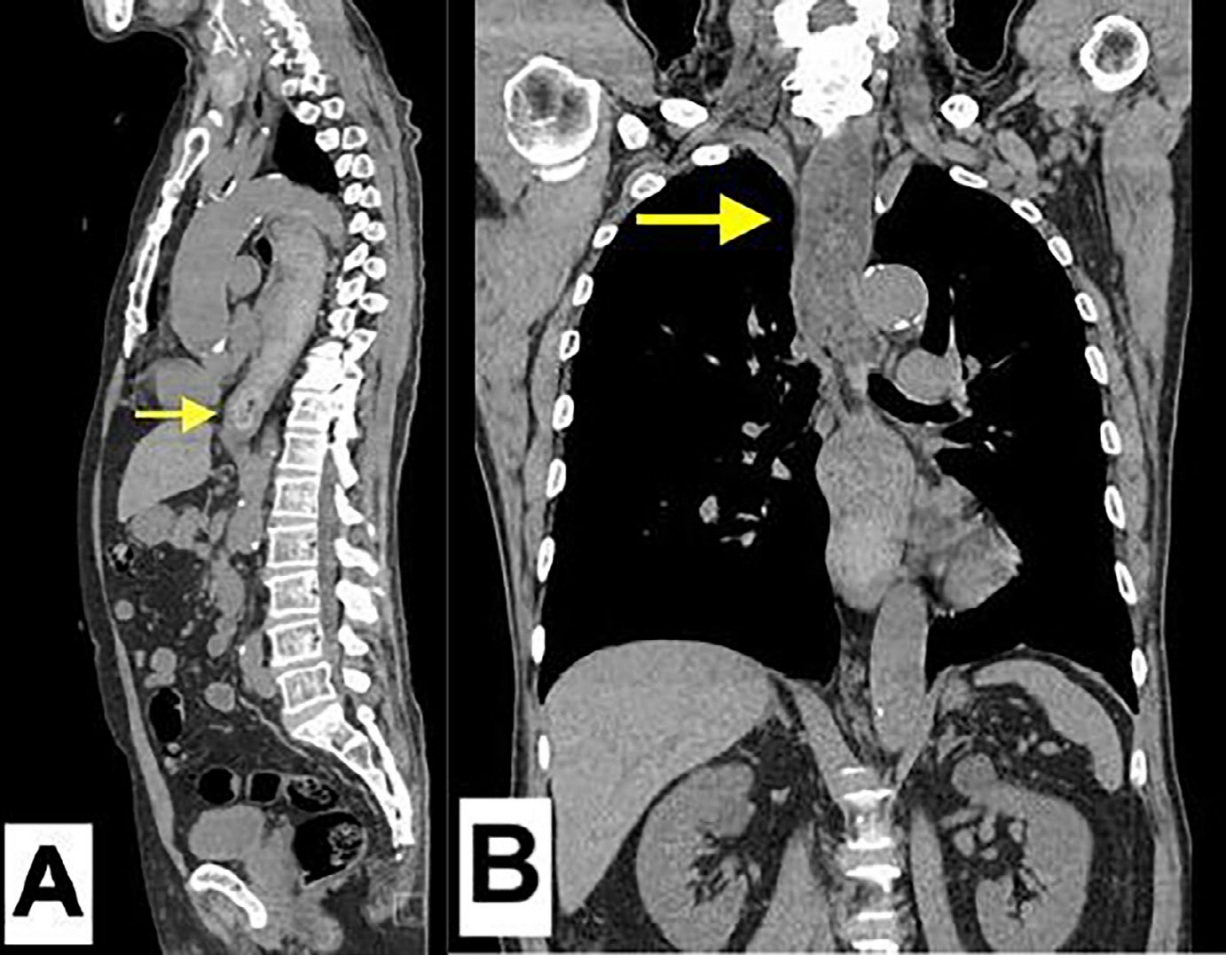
CT images of esophageal obstruction caused by octopus ingestion. (A) Axial CT scan shows an obstruction at the esophagogastric junction. The yellow arrow identifies an octopus fragment lodged at the esophagogastric junction, resulting in partial obstruction. (B) Coronal CT scan shows retained food residues, as indicated by the yellow arrow, caused by an obstruction at the esophagogastric junction and extending proximally to the cervical esophagus.
